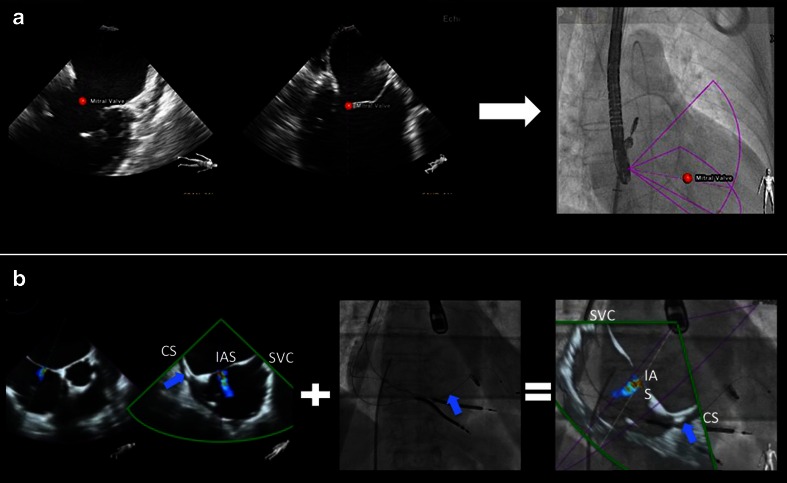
Tools in fusion imaging: markers (a) and overlay imaging (b). During the MitraClip intervention, the main mitral lesion can be labeled by the echocardiographer using a marker (*red dot* placed on the mitral valve). This marker is automatically demonstrated in real time on the fluoroscopy image (a). Using the overlay mode (b, showing an atrial septal defect), the echocardiographic image is overlaid on top of the fluoroscopy image in the correct position and angulation. The interventional cardiologist can choose between the purple and the green echo views given by xPlane echocardiography. In this case, the *green* view better demonstrates the anatomy for puncture purposes. The overlay mode highlights the orientation difficulties that SHD teams face during an intervention. In the modified TEE bicaval view, the superior vena cava is located to the right, while the coronary sinus is to the left, connected by the horizontally orientated interatrial septum. During fluoroscopy for transseptal puncture, the orientation of the C-arm is mostly neutral (in our example, LAO 13.5°, CRAN 0°). This leads to a completely different orientation: the superior vena cava is cranial, while the coronary sinus is at the bottom and to the lateral, with a more or less vertical interatrial septum. *SVC*, superior vena cava; *CS*, coronary sinus; *IAS*, interatrial septum. *Blue arrow*: depicting the left ventricular lead in the CS. a Adapted from Sündermann et al. [32••]
